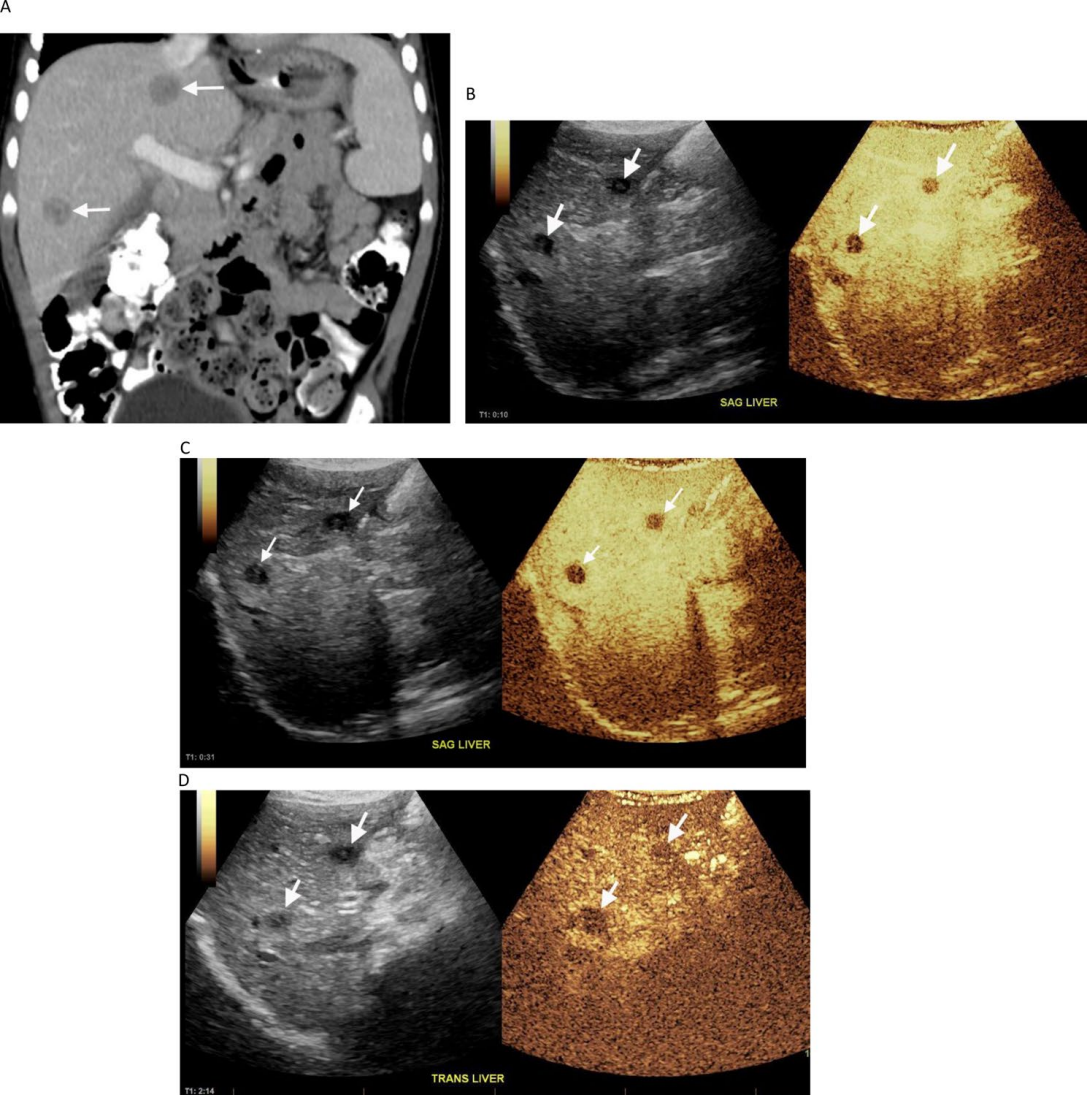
2-year-old male with a presacral yolk sac tumor developed multiple FLL’s while on therapy. (A) Coronal contrast enhanced CT image shows two well defined hypodense lesions (arrows). (B) Arterial-phase CEUS image obtained at 10 s after contrast administration shows two hypoenhancing lesions (arrows). (C) Portal-venous-phase CEUS image obtained at 31 s after injection and (D) delayed-phase CEUS image obtained at 2 min and 14 s after injection again show hypoenhancement of the lesions (arrows). These were pathologically proven to be abscesses with necrotizing granuloma
